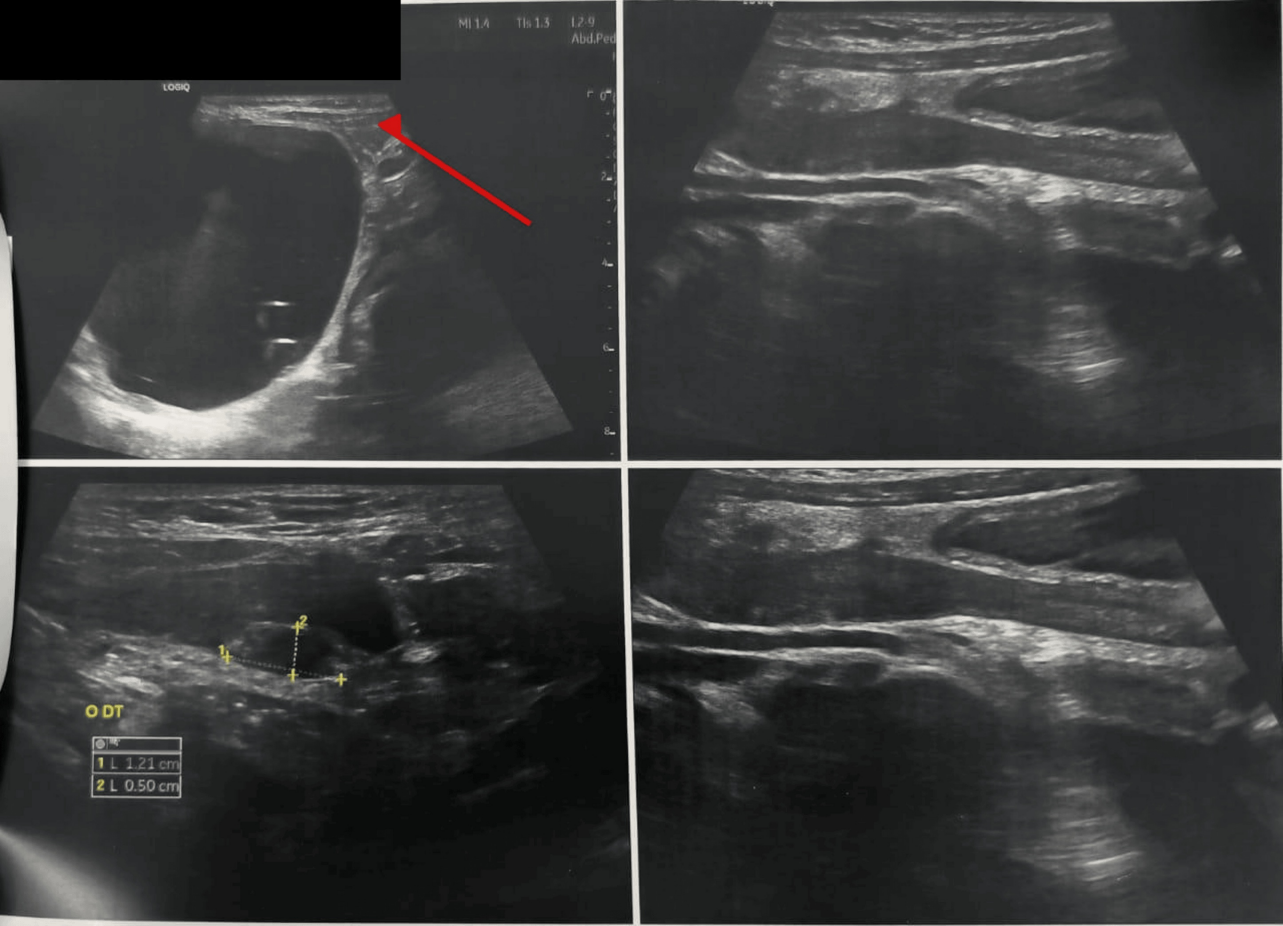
Abdominal ultrasound showing a large cystic abdominal mass occupying most of the abdominal cavity, suggestive of an adnexal origin (red arrow) Red arrow: cystic mass on the left side
